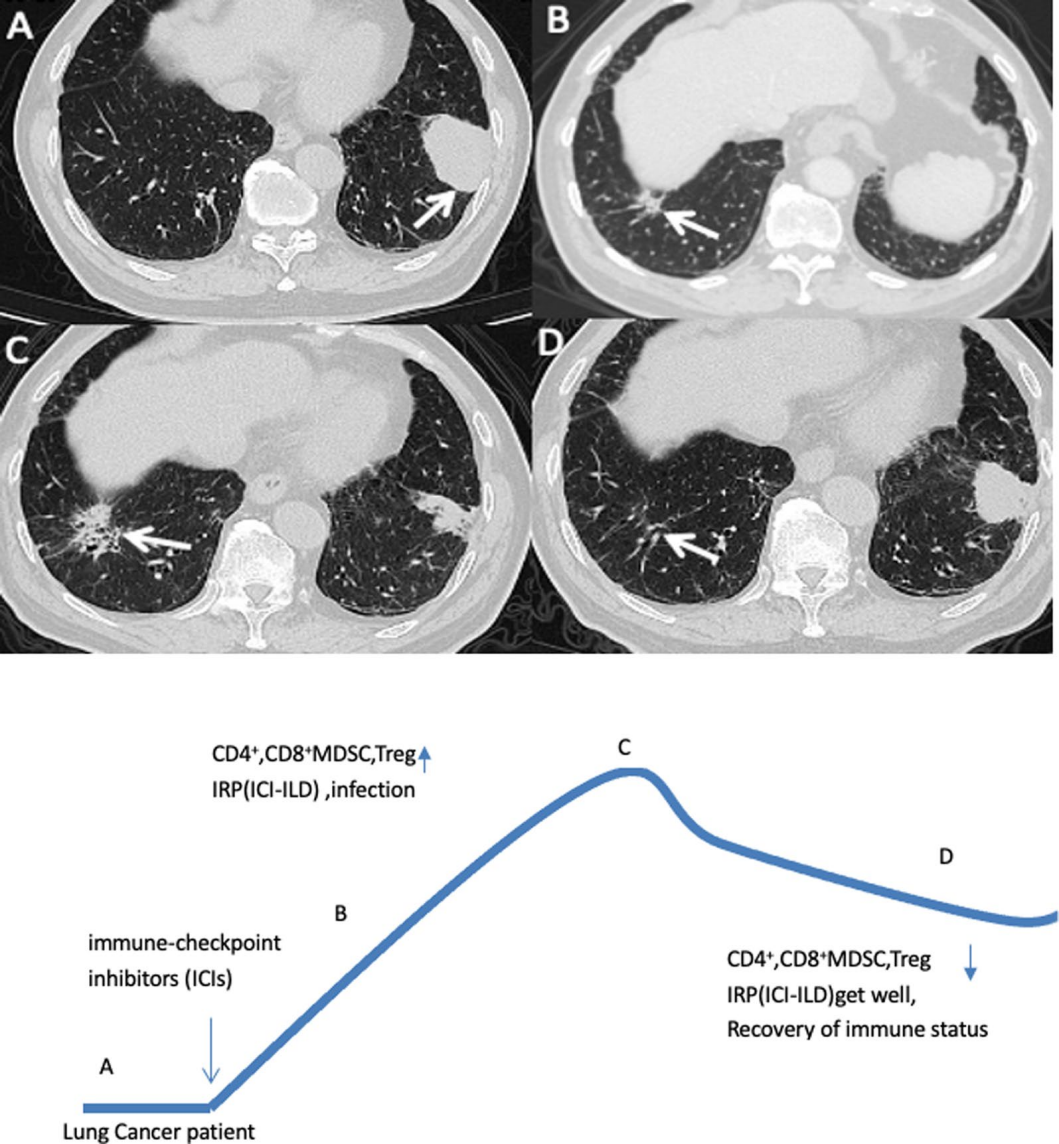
Computed tomography (CT) images during the clinical course of the patient. A lung adenocarcinoma of the left lower lobe (arrow), B new lesions of the right lower lobe (arrow), C progress of new lesions of the right lower lobe (arrow), partial remission of the lung adenocarcinoma of the left lower lobe, D regression of lesion of the right lower lobe (arrow), E Mechanism of immune-related pneumonitis caused by immune reconstitution inflammatory syndrome
